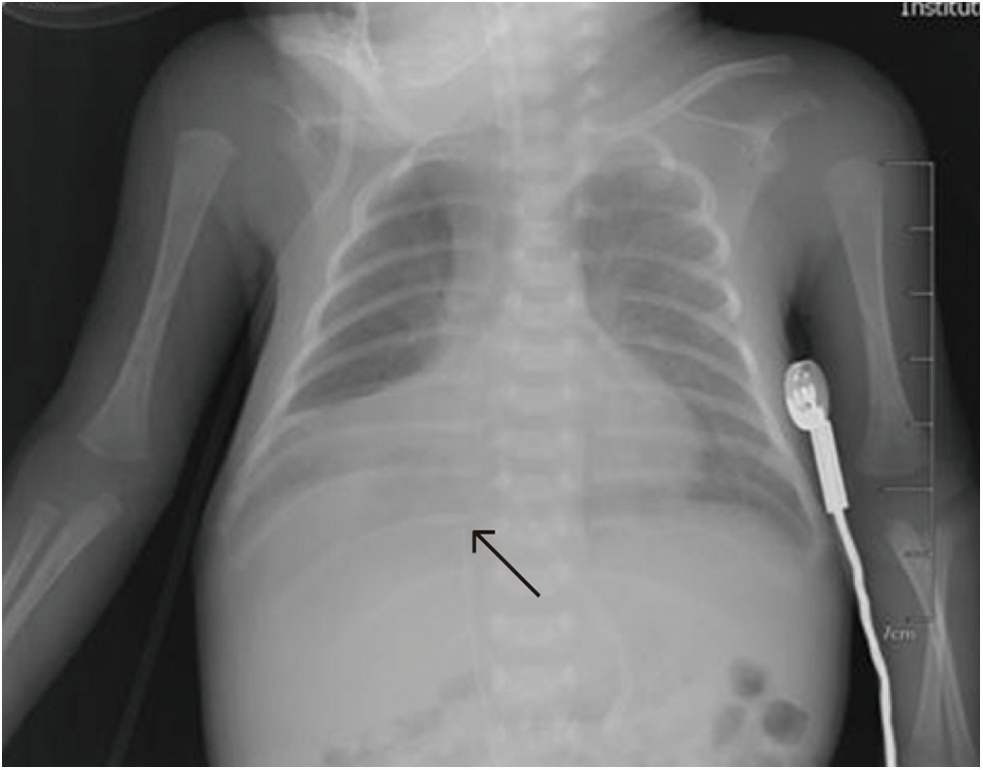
In patient N, the catheter was inserted in the great saphenous vein, and chest X-ray shows that it had reflexed. After correction, it was located at T10, and X-ray revealed pleural effusion (PE) 7 days later.
